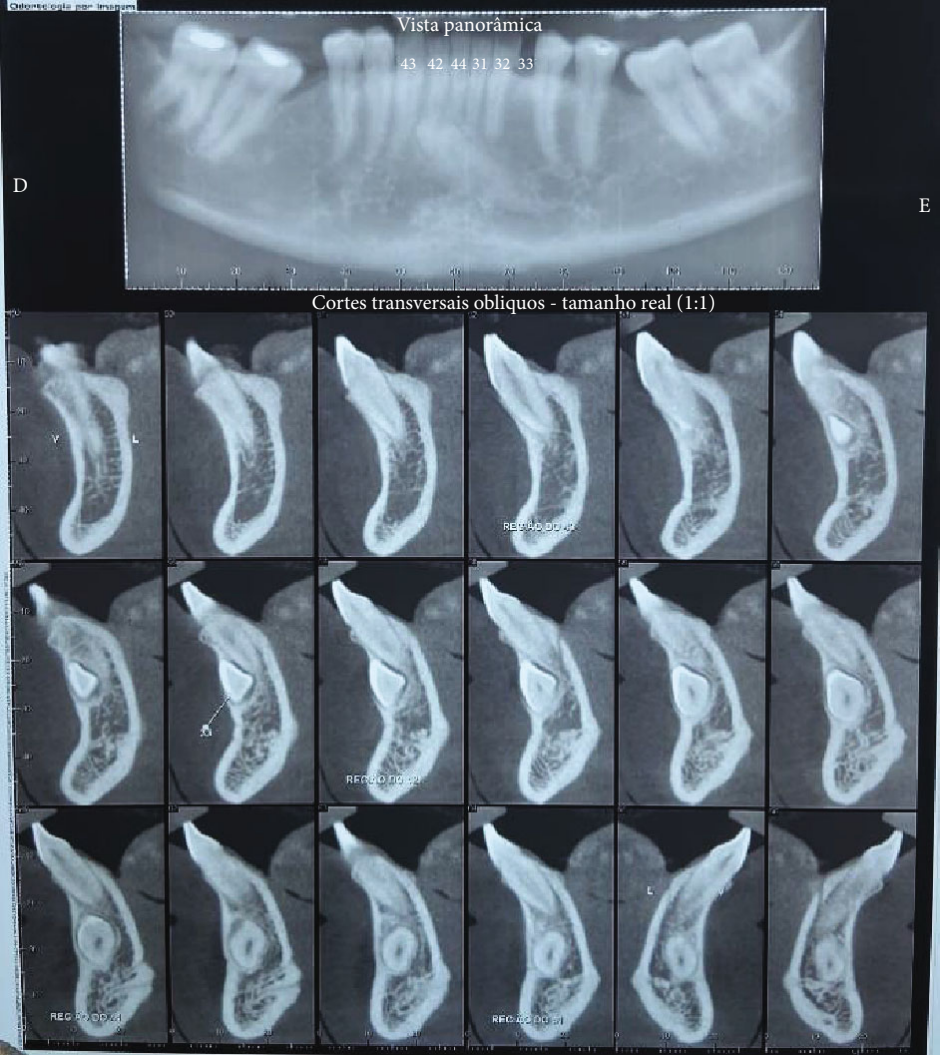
Computed tomography (CT) scan (panoramic view and oblique cross-sections).
